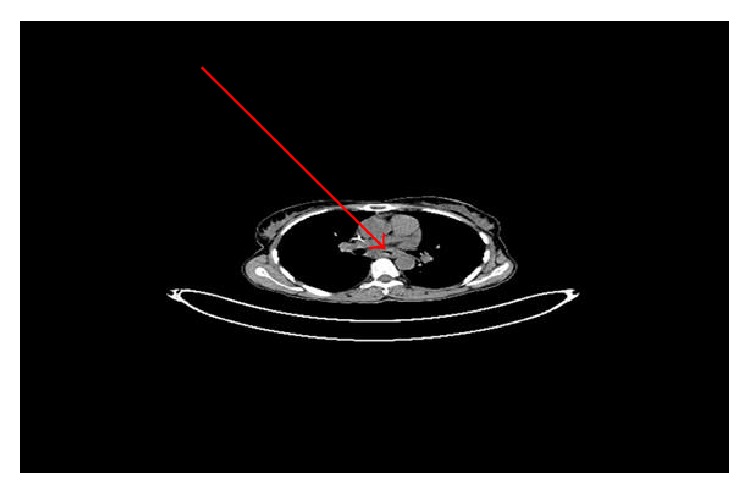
After neoadjuvant therapy, control CT of regressed mass (arrow).
